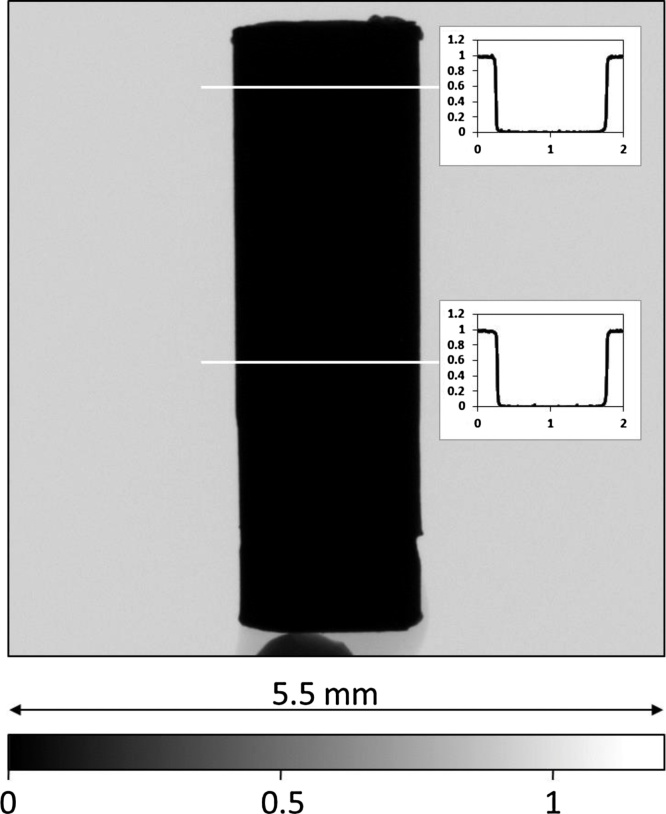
X-ray radiograph of the gold sample with artificial pore space. The inserted transmission profiles confirm that hardly any X-rays are transmitted through the sample.
